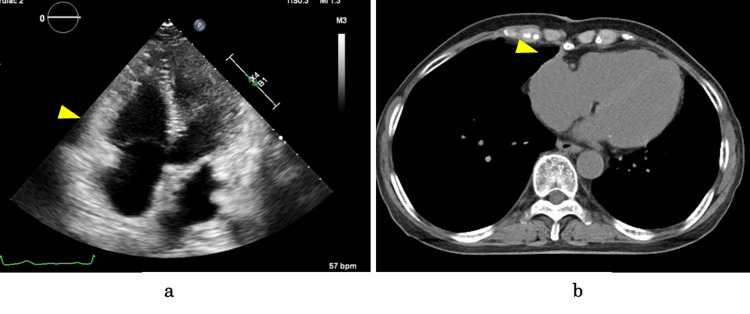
Postoperative echocardiography and computed tomography Postoperative echocardiography demonstrating (a) the resolution of cardiac compression (arrow) and improvement in the right ventricular function and (b) the absence of residual mass or recurrence (arrow).
